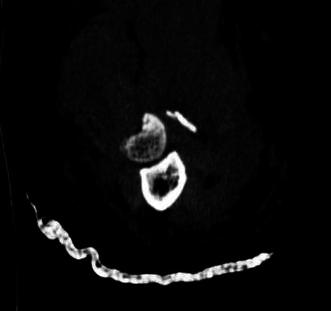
CT scan of unicondylar radial head fracture. Example of an axial cut that best revealed the largest diameter of the intact portion of the radial head and associated fracture defect used to quantify the fracture
